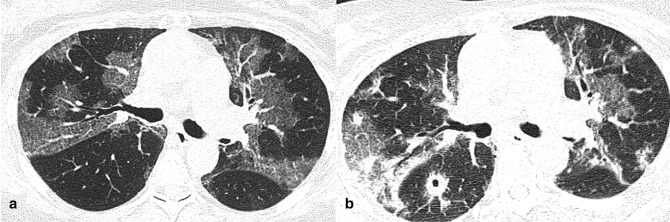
Evolution of CT findings in a 56-year-old female COVID-19 patient (δ variant). (a) CT scan, performed after 3 days from symptom onset, showed GGO with superimposed inter- and intralobular septal thickening (crazy-paving pattern) in upper lobes. (b) Scan obtained on day 7 from symptom onset, showed peripheral consolidations. In apical segment of right lower lobe, CT demonstrated rounded consolidation with cavitation in context.
